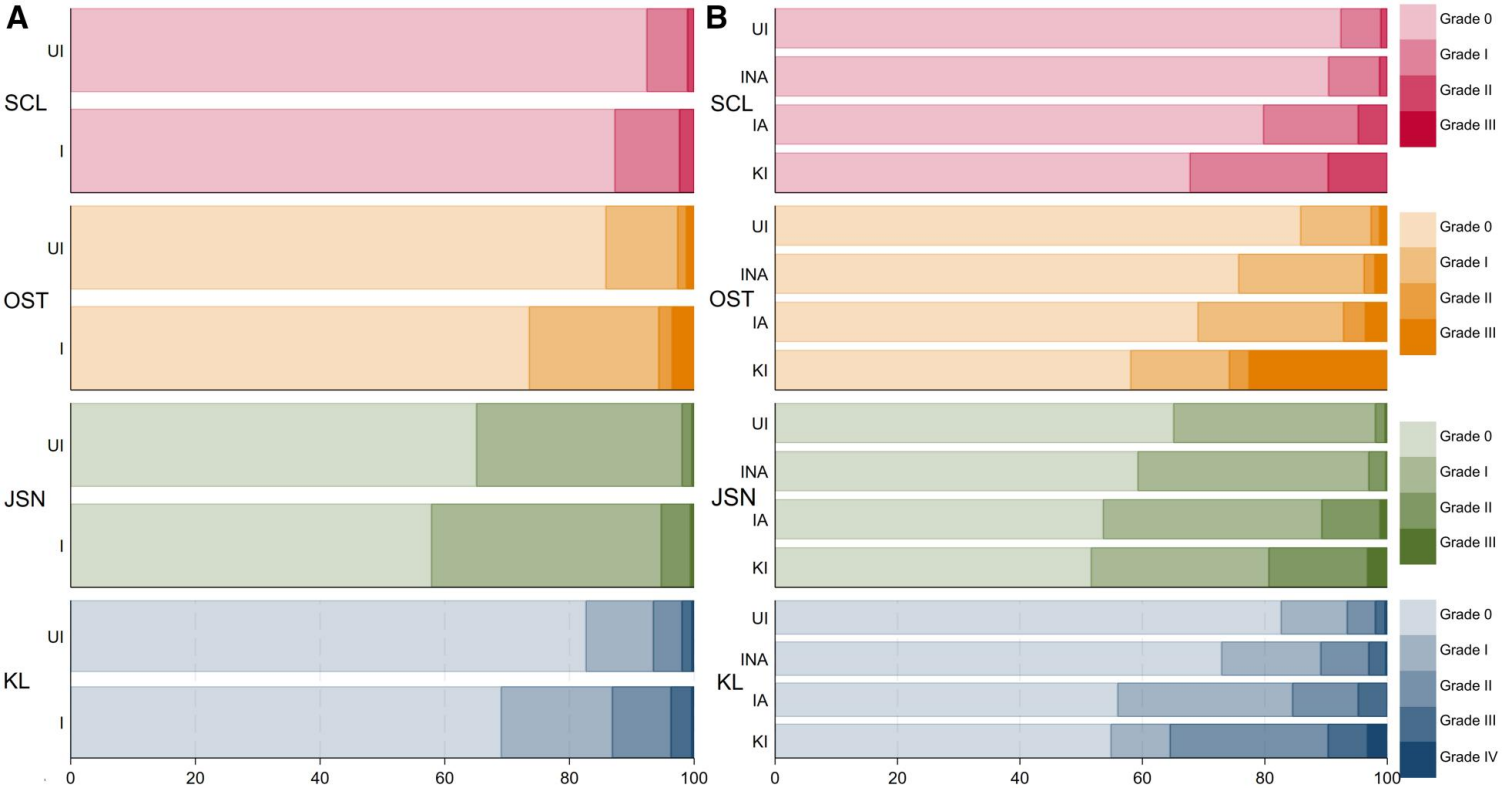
Radiographic results for (A) uninjured and injured and (B) UI, INA, IA and KI. Participants percentage of each grade for each imaging subscale. (A): *n* = 564 and 510, (B) *n* = 564, 395, 84, and 31, respectively. OST: osteophytes; SCL: sclerosis.
